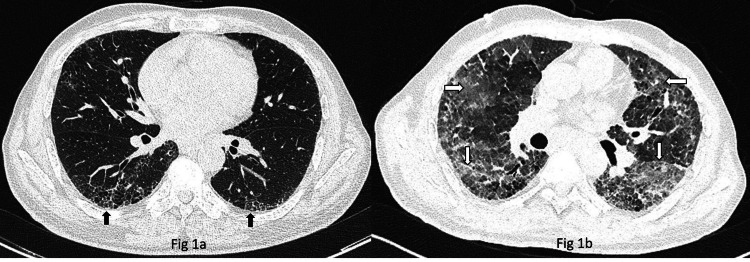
Rituximab-induced interstitial lung disease (a) Computed tomography scan of thorax shows bilateral basal reticulations and subtle honeycombing changes (upward arrows) consistent with a possibility of a usual interstitial pneumonia pattern. (b) Computed tomography scan of thorax shows extensive GGOs (horizontal arrows) and interlobular septal thickening (downward arrows) consistent with findings of acute interstitial pneumonia. GGOs - Ground Glass Opacities
